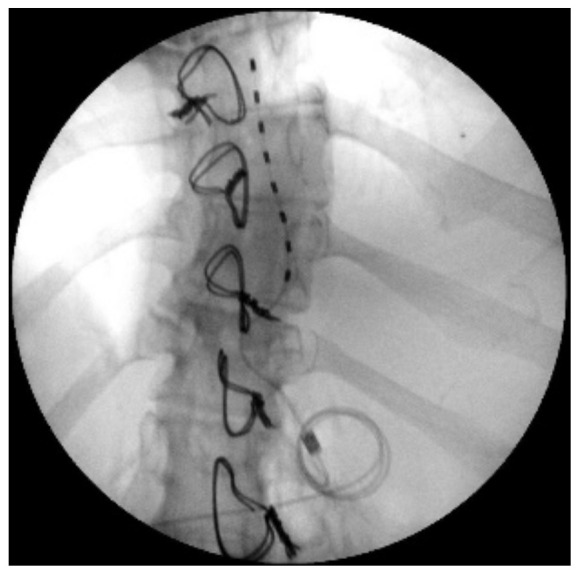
X-ray image of loop for subcutaneous fixation.
